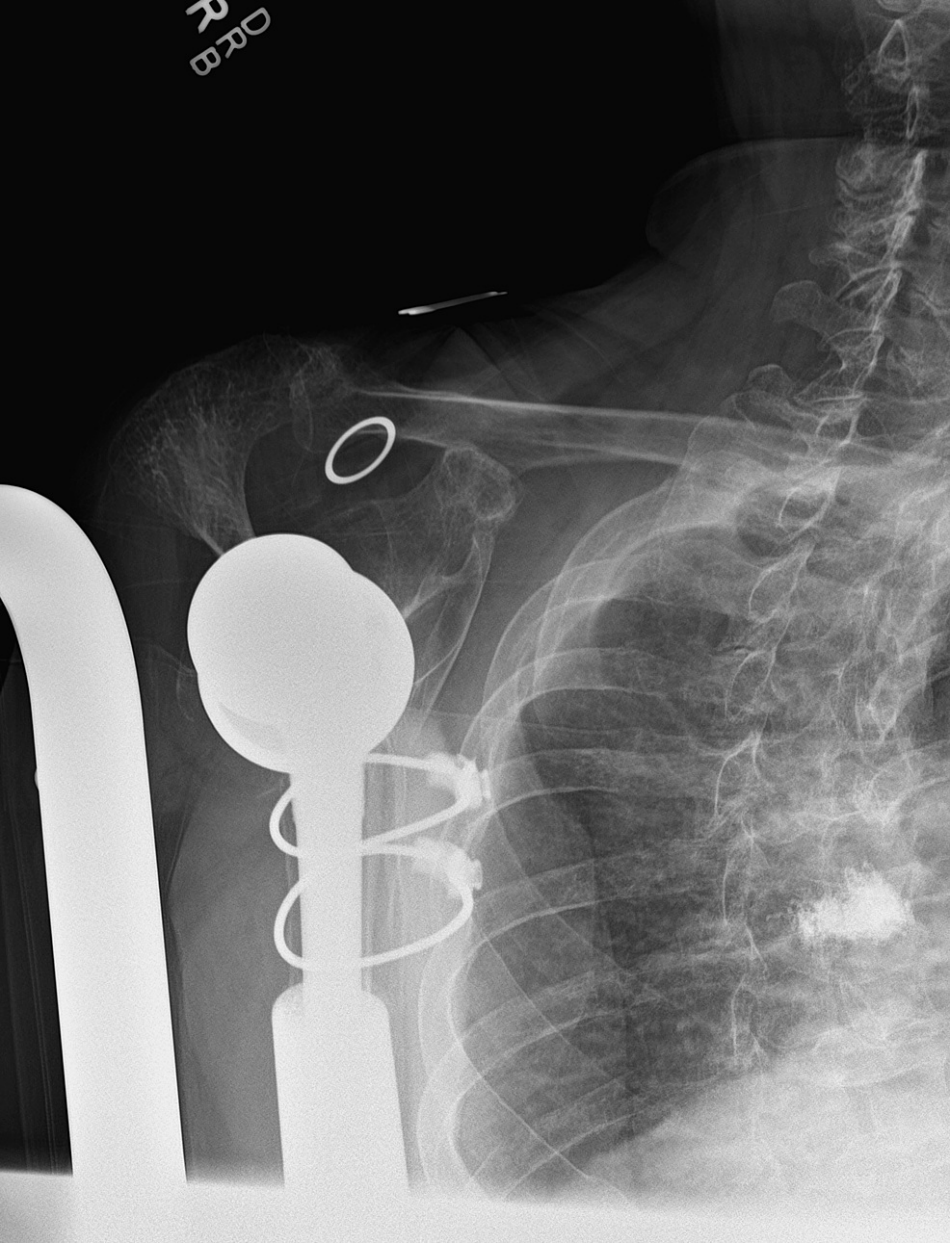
Lateral radiograph of the right shoulder at 12-month follow-up.
